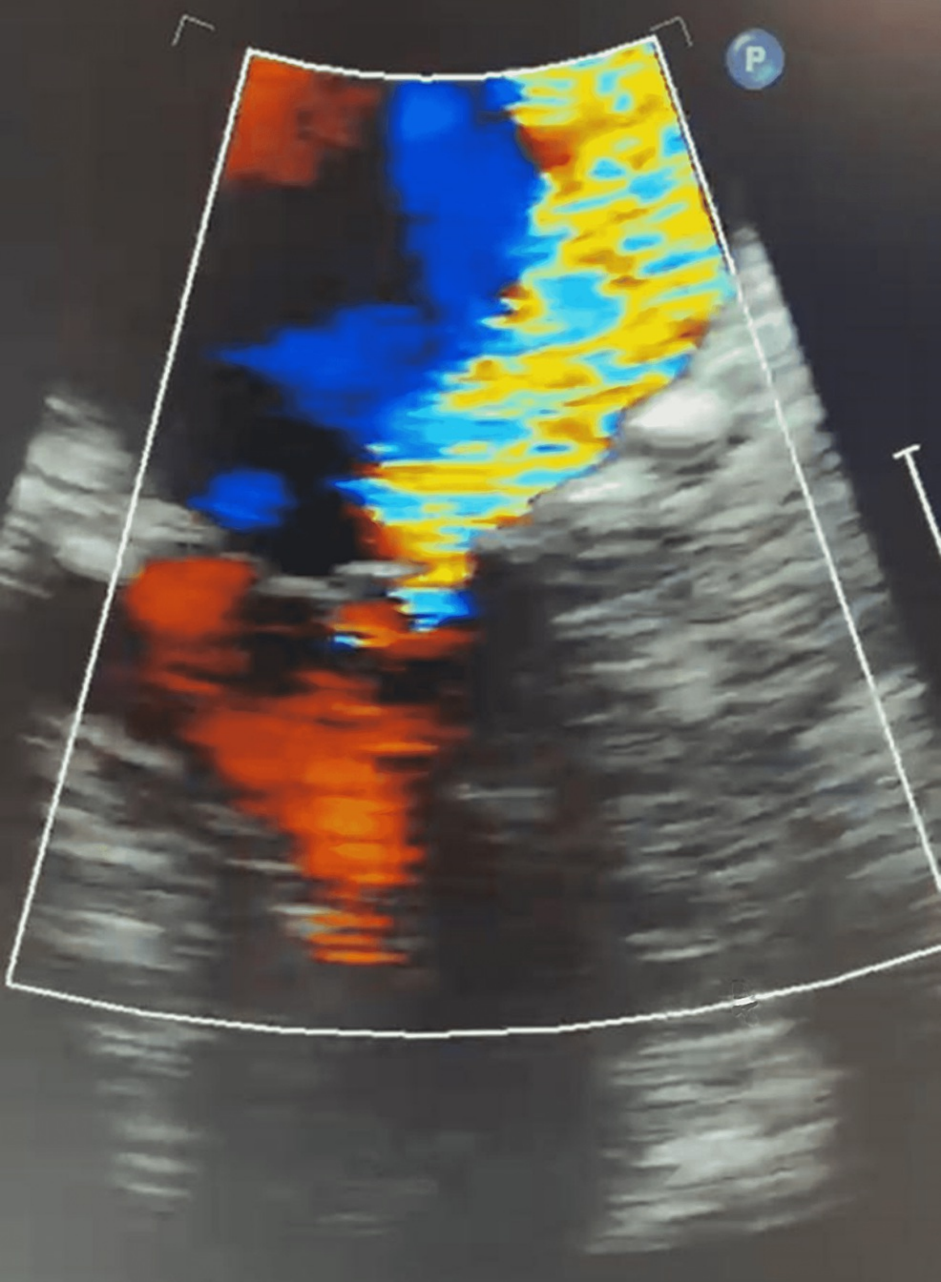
Close-up transoesophageal echocardiogram demonstrating posteriorly directed eccentric jet of severe mitral regurgitation.
